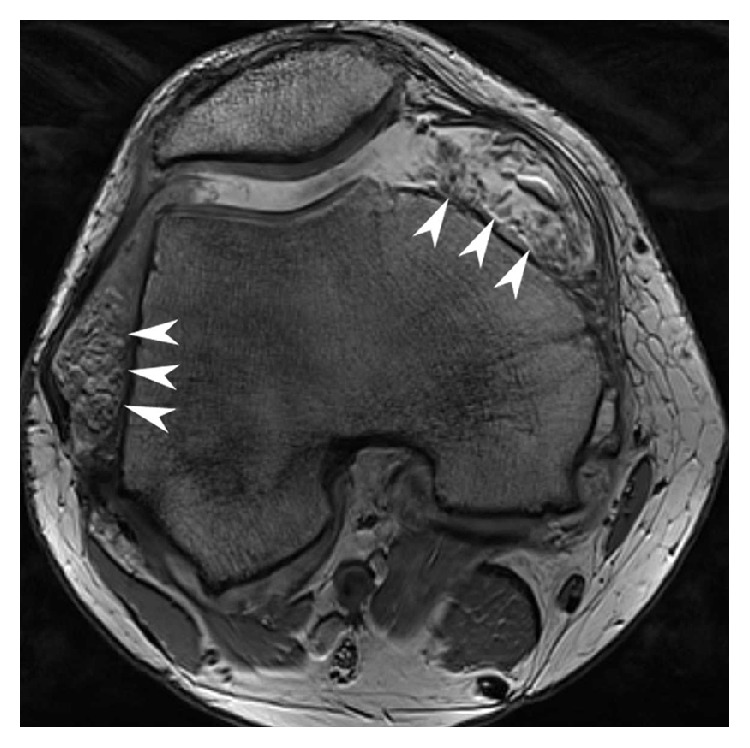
Transverse T2-weighted gradient echo (DESS 3D) image through the patellofemoral joint clearly depicts extensive synovial proliferations in the medial and lateral recess (arrowheads).
